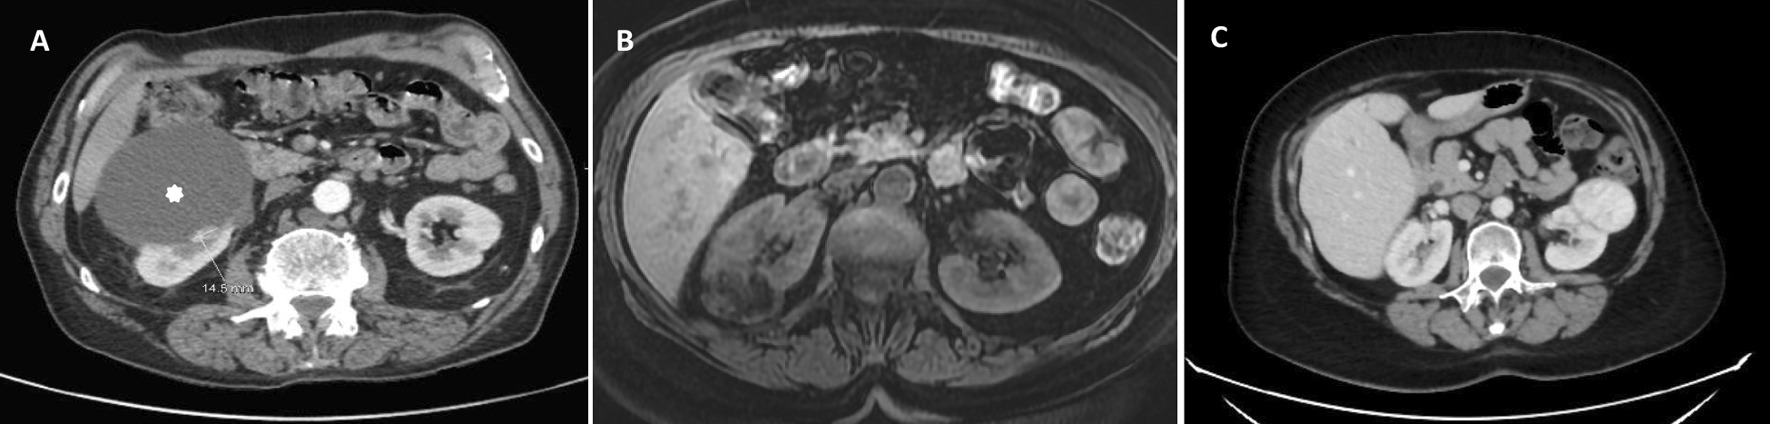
Abdominal computed tomography scan showing a Bosniak 4 cystic lesion (white star) and a fleshy lesion (white line) of right kidney in Case 1 (A), a right mid-renal lesion with fat and tissular components in Case 2 (B) and a left kidney mass in Case 3 (C)
